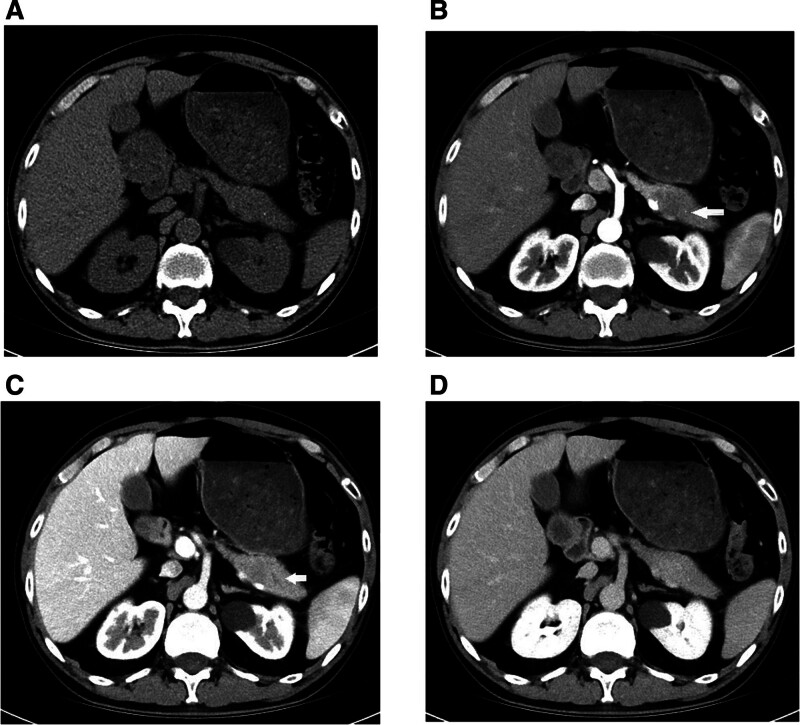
Contrast-enhanced abdominal CT images demonstrating a pancreatic tail mass (arrows) with gradual enhancement. (A) Non-contrast phase; (B) arterial phase; (C) portal venous phase; (D) delayed phase. CT = computed tomography.
